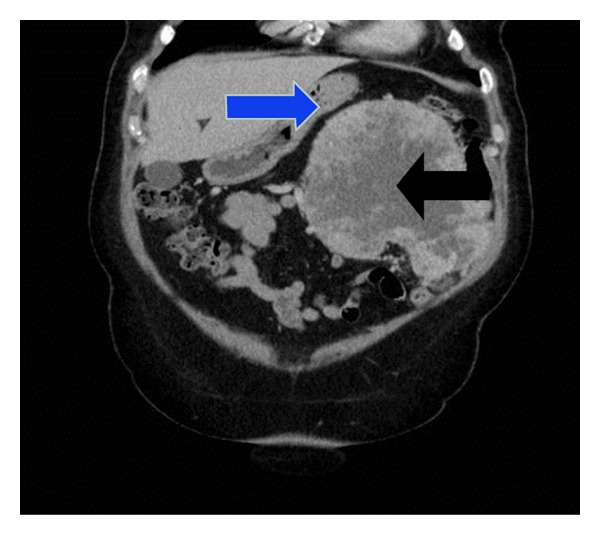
Computerised tomography (CT) scan of abdomen showing the renal mass (black arrow) close to the stomach (blue arrow).
